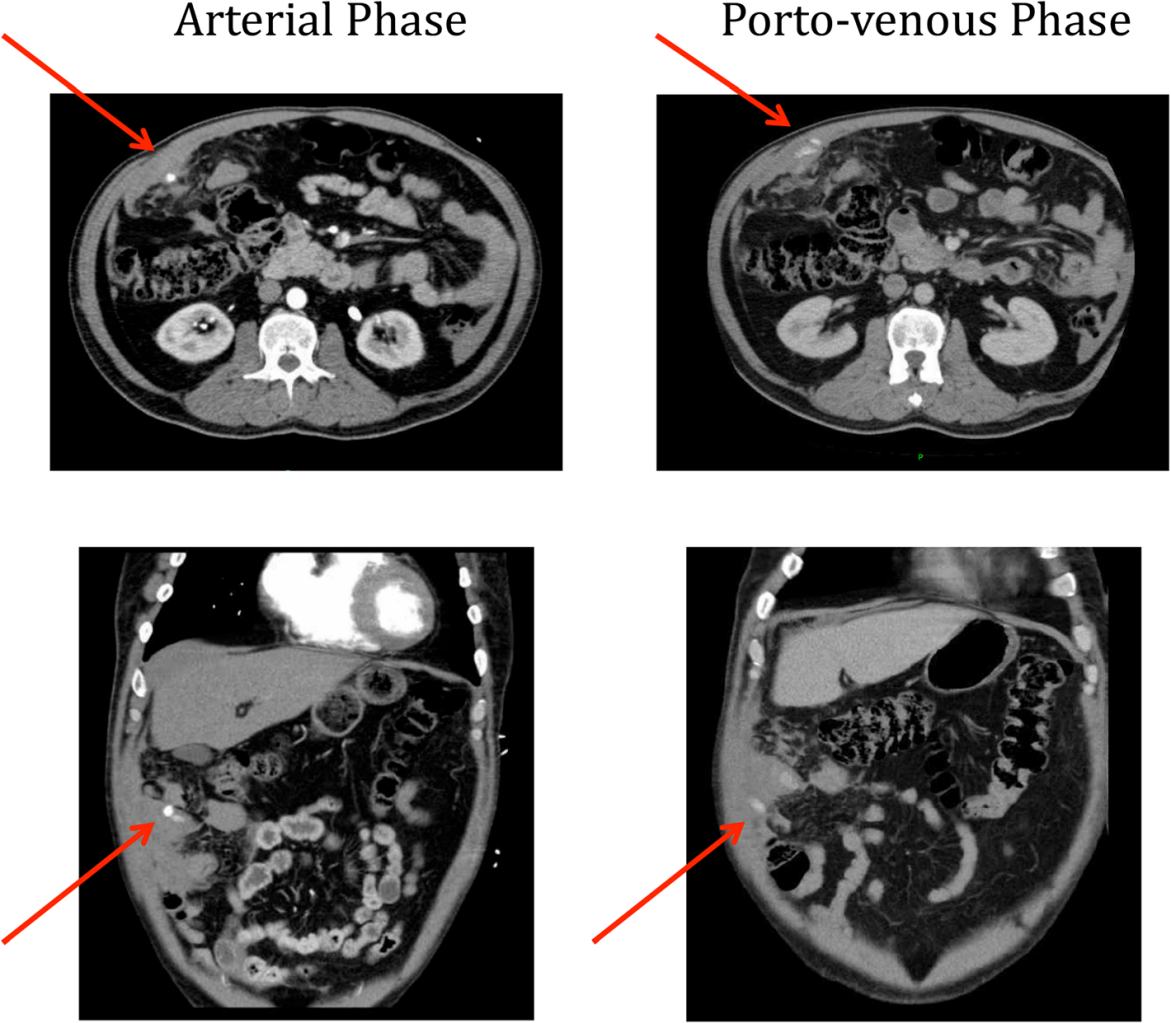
CT abdomen/pelvis with IV contrast with arterial and porto-venous phases demonstrating blush (*arrows*) and free high attenuation fluid in the peritoneum
